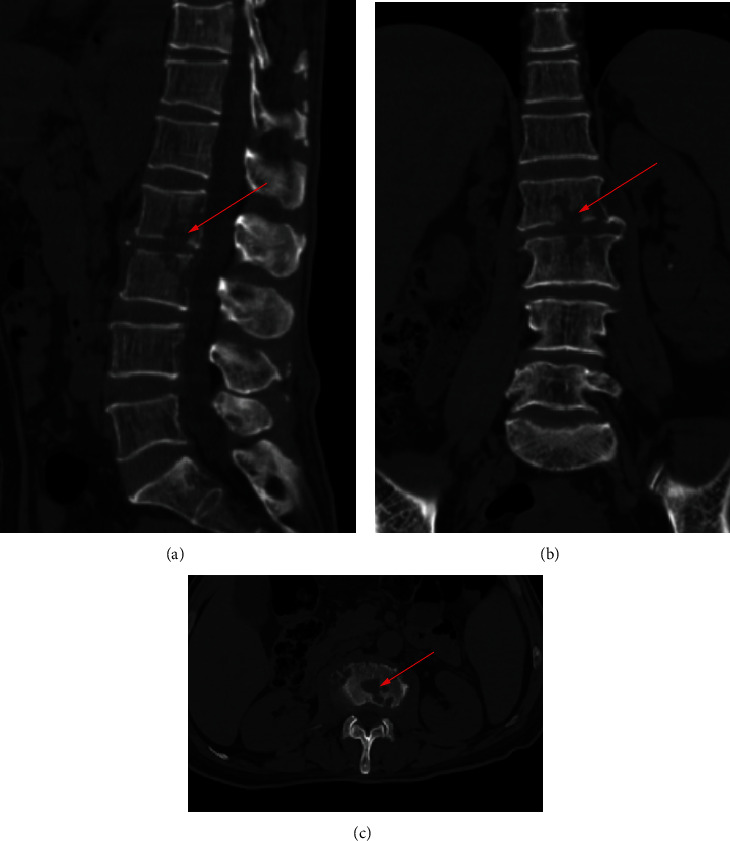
CT examination of a patient with spinal tuberculosis. The arrow points to the location of the lesion. (a) CT image in sagittal position. (b) CT image in coronal position. (c) CT image in cross-section.
